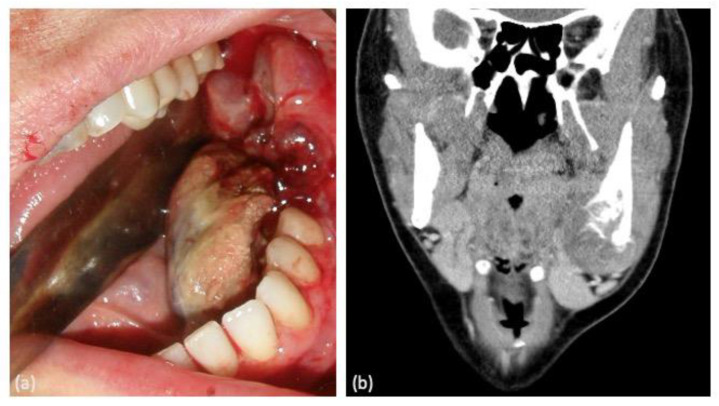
(a) Intraoral clinical manifestation of the tumor in the left mandibular molar area showing bleeding. (b) Computed tomography coronal section image of the same lesion showing an area of osteolysis.
